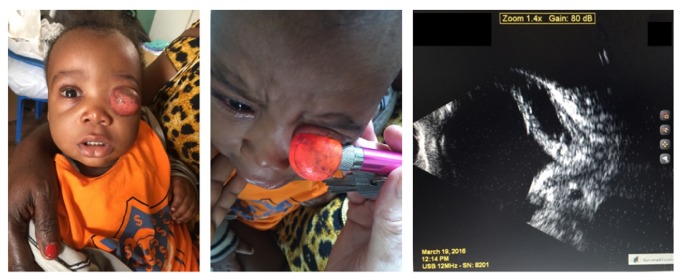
Patient with a clinical anophthalmos with orbital mass in the left orbit. A: protruded cyst. B: transillumination with a pen light revealed the cystic character of the structure. C: B-scan ultrasonography examination.
